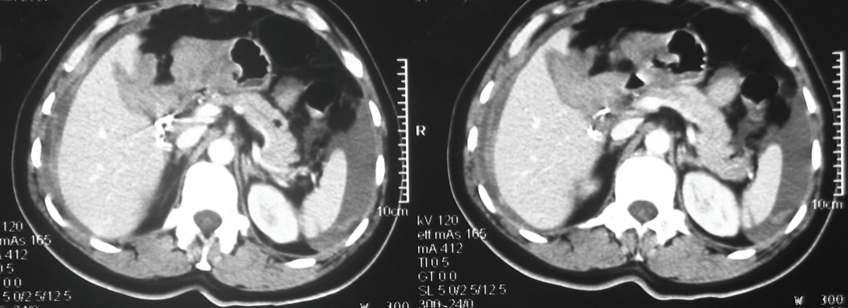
CT scan showing contrast extravasation (grade III splenic fracture); perisplenic and perihepatic hemoperitoneum.
